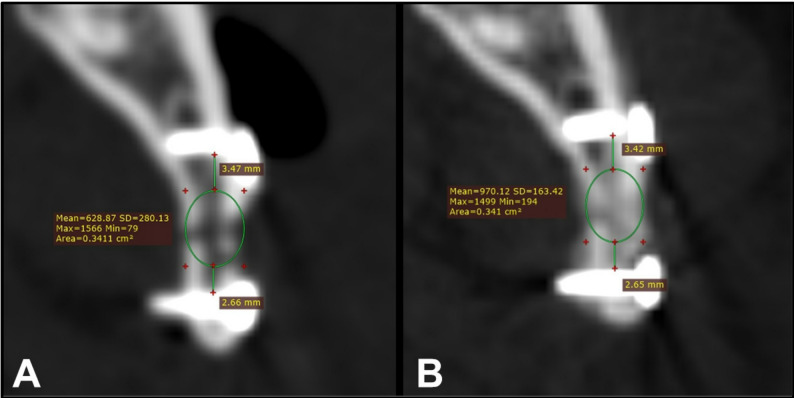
Axial view, CT scan analysis of bone density in HU using RadiAnt® DICOM Viewer software. A) Immediate post-operative CT scan analysis of bone density in HU at region of interest delineated over the fracture site. B) 12 weeks follow-up CT scan analysis of bone density in HU at region of interest delineated over the fracture site
